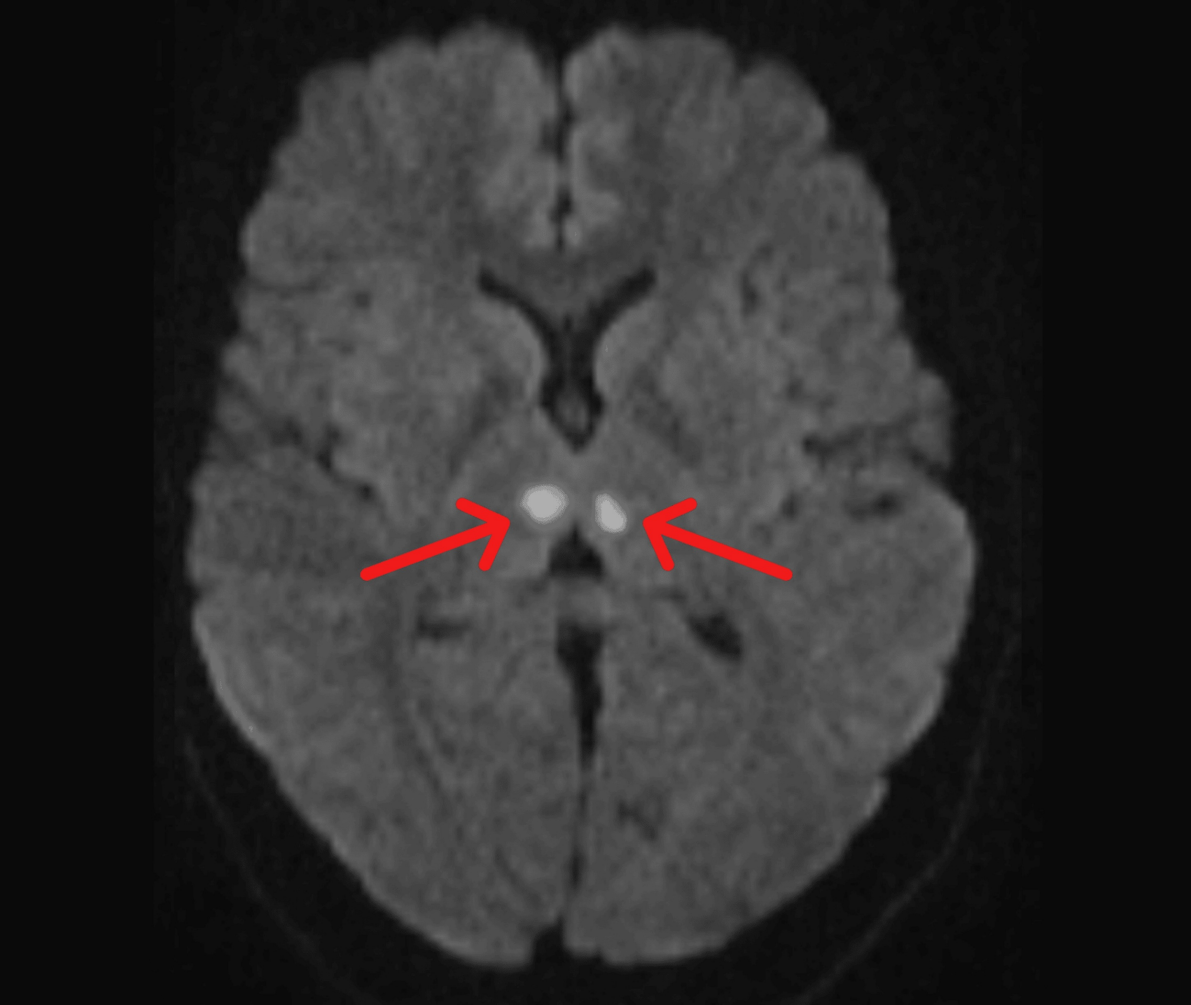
Axial FLAIR at the level of basal ganglia showing FLAIR hyperintense focus in bilateral medial thalami FLAIR: fluid-attenuated inversion recovery
